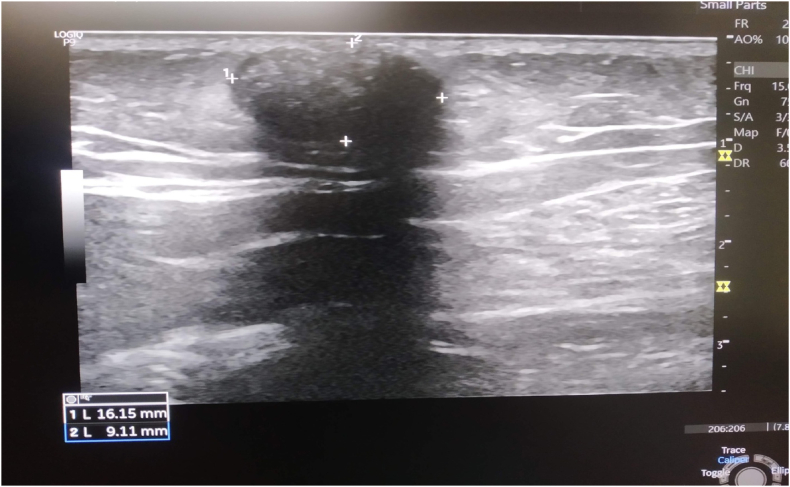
Ultrasonography showing a well-defined, oval, hyperechoic lesion with posterior acoustic shadow in the subcutaneous plane of the left buttock.
